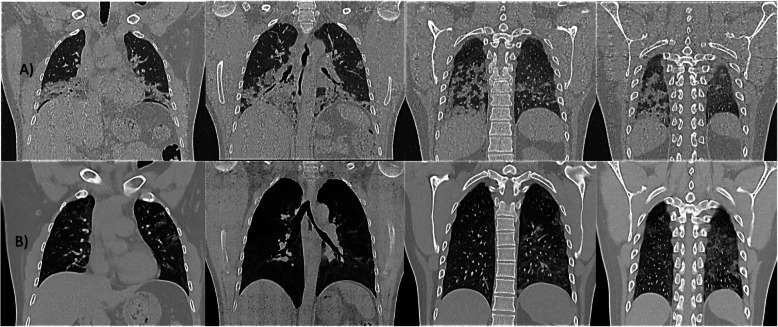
Case 5: A 42-year-old male, without comorbidities, score 4 at the moment of the recruitment, with oxygen requirements through a nasal cannula. High-resolution CT chest: a day of admission (April 1st). There are right lung lower lobe and middle lobe extensive consolidations. Similar peripheral opacities noted in the left lung lower lobe and upper lobe. b Day 4 after treatment (April 13th, nebulization did on April 9th). Significant improvement of previously noted patchy consolidations at bilateral lower lobes and right middle lobe, as well as few peripheral consolidations in the left upper lobe
